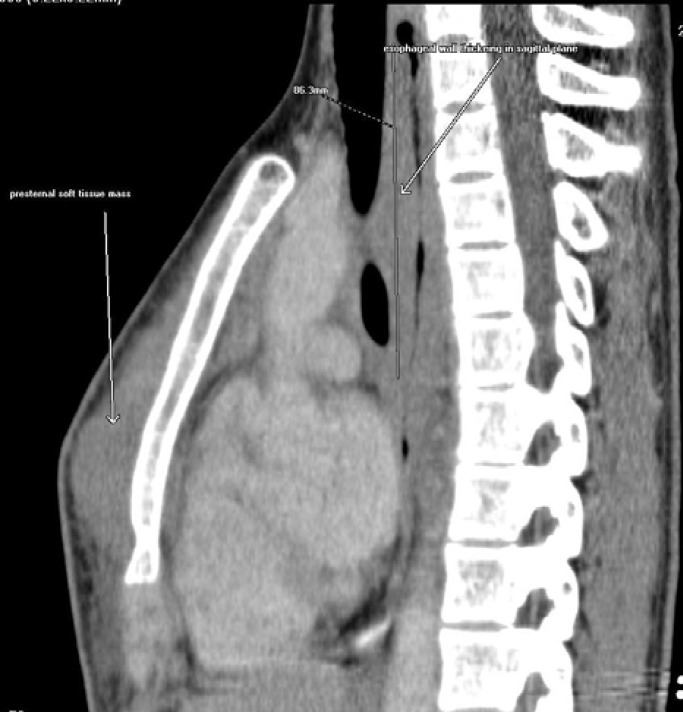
Sagittal CT image shows gross circumferential thickening with luminal narrowing involving upper and mid esophagus with a soft tissue mass lesion of size 10×6×2cm in anterior chest wall in left para sternal region Figure 3. Sagittal CT image shows gross circumferential thickening with luminal narrowing involving upper and mid esophagus with a soft tissue mass lesion of size 10×6×2cm in anterior chest wall in left para sternal region
